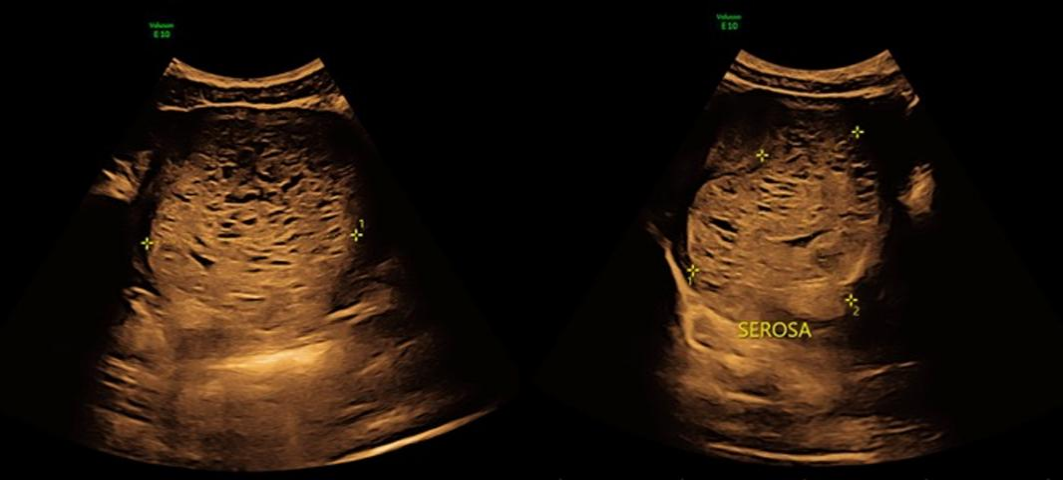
Ultrasound image of the 10-cm molar pregnancy invading the myometrium.
